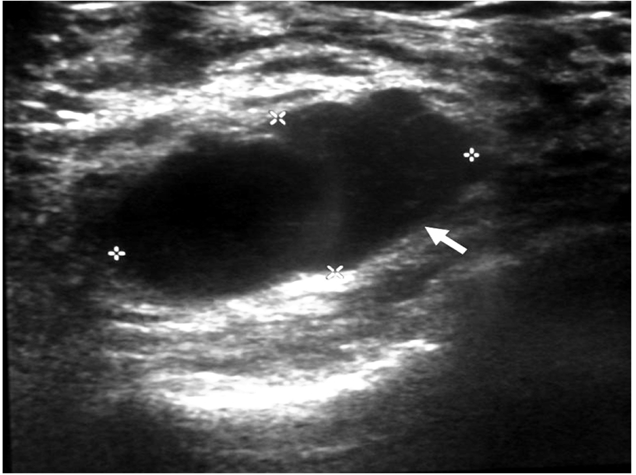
Ultrasound examination of the right breast showing a well circumscribed hypoechoic mass (white arrow) with internal echogenicity and acoustic enhancement with lobulated margins.
